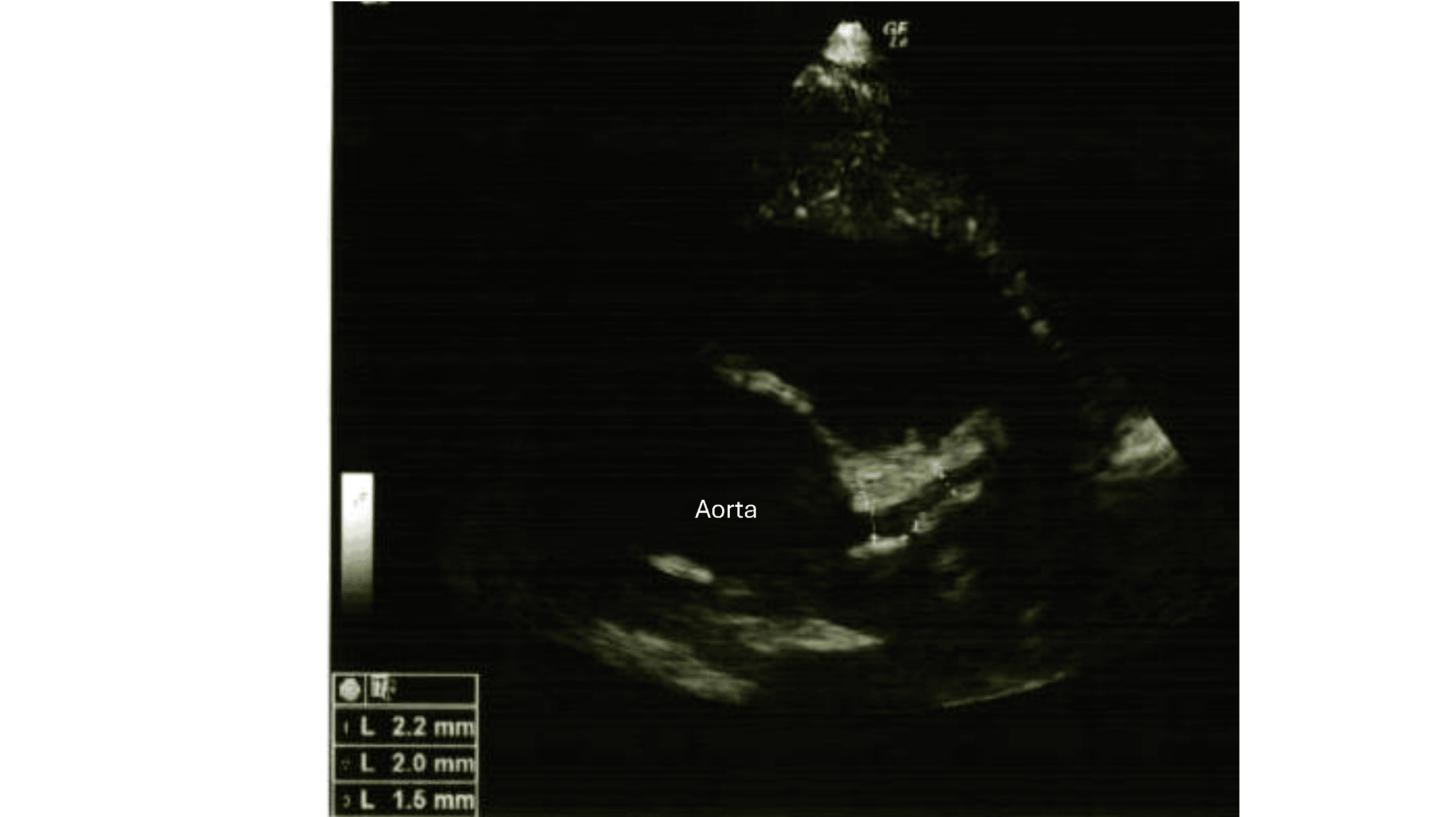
Echocardiography at admission. Echocardiography revealed no cardiac involvement, including coronary arterial lesions.
